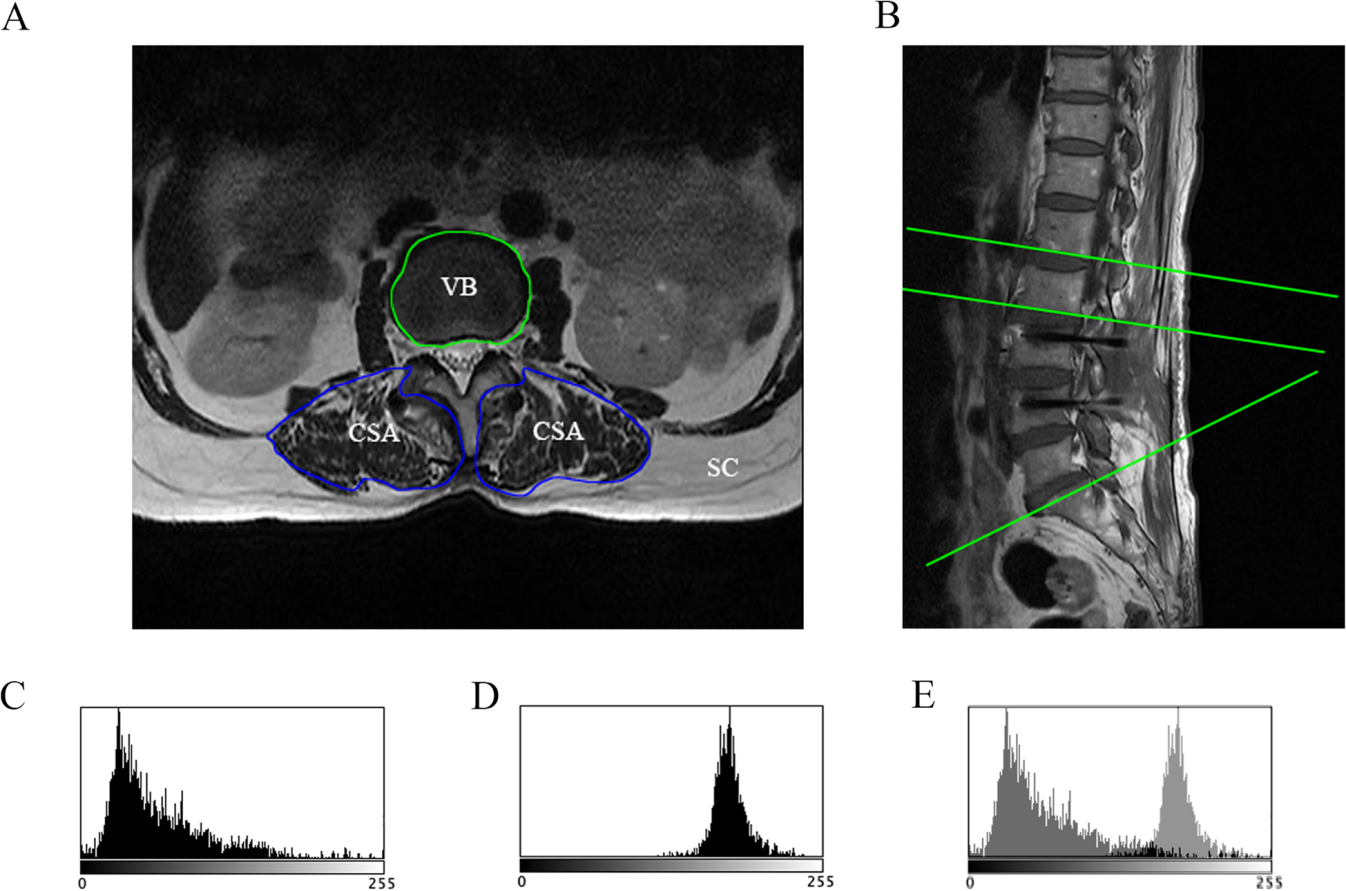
Mesurement of the multifidus muscle: a: T2-weighted axial slices of the lumbar spine b: the selection of the slices; c&d: The gray-scale range of the signal intensity histogram was measured within the cross-sectional area (c) and subcutaneous fat (d); e,: The grayscale value of the cross-sectional area region overlap with the subcutaneous fat region
